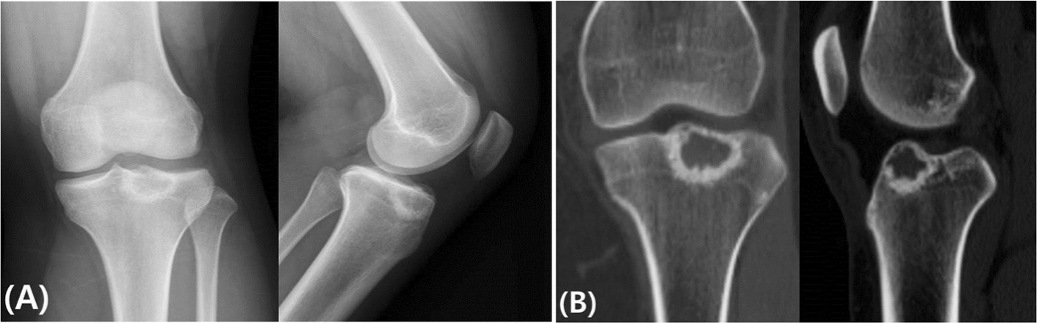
Plain radiographic images and computed tomograhic scan. (A) Anteroposterior (left) and lateral (right) radiographs show the proximal tibial epiphyseal osteolytic lesion with marginal sclerosis and a matrix with a ground glass appearance and without periosteal reaction. (B) Coronal (left) and sagittal (right) computed tomographic scans show the absence of cartilage matrix and dense marginal sclerosis without cortical disruption.
